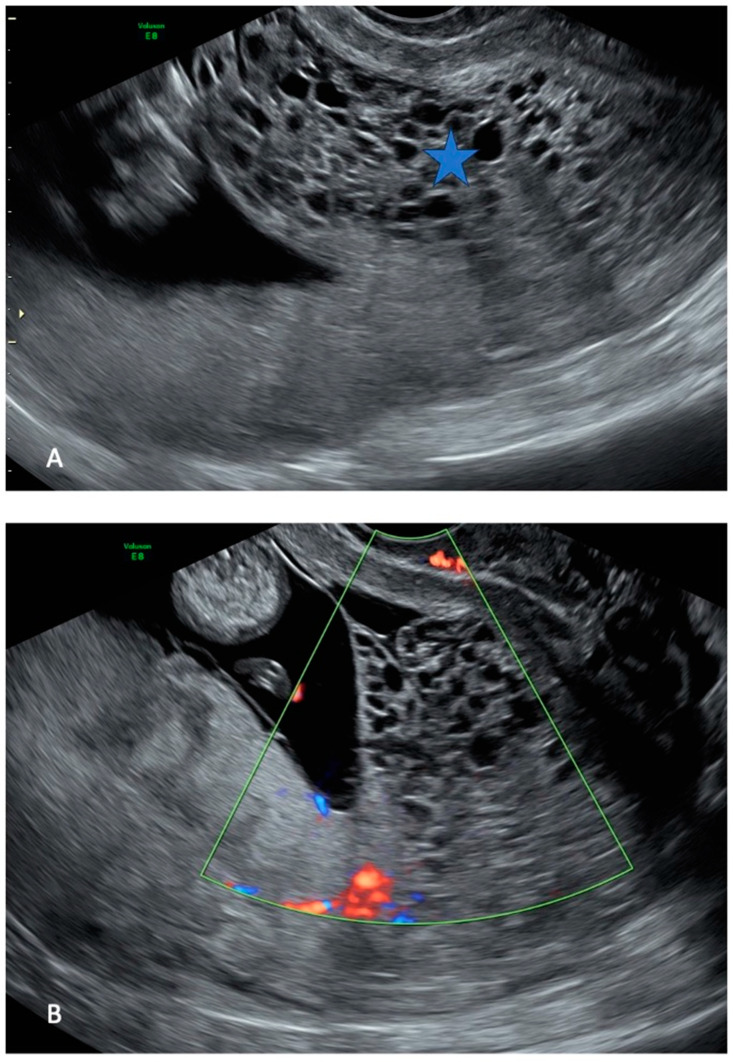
Ultrasound images of hydatiform mole at 13 weeks of gestation: a normal intrauterine pregnancy ((A,B) left side) coexisted with a multicystic area ((A) star, (B) echographic cone), compatible with molar degeneration.
